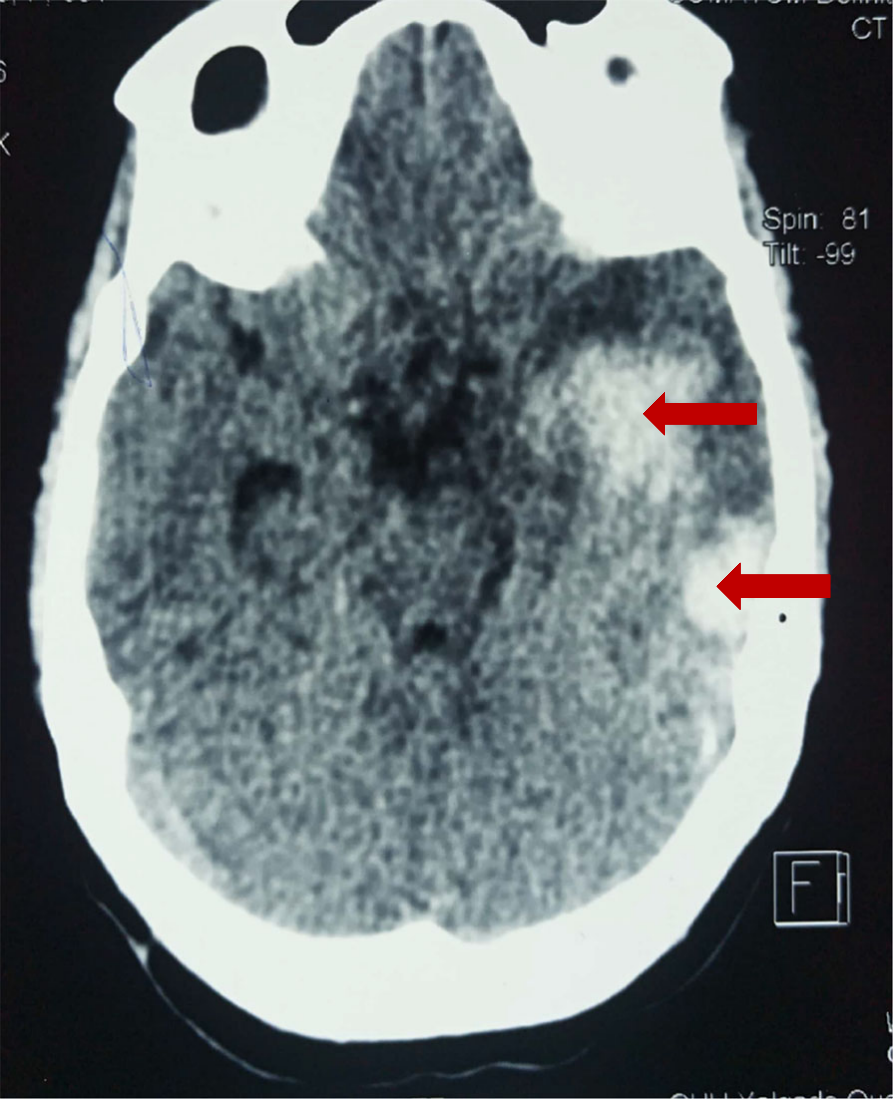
Brain CT showing right spontaneous temporal hematoma, in a 30-year-old woman presenting with motor deficit, speech disorder, and consciousness disorder
